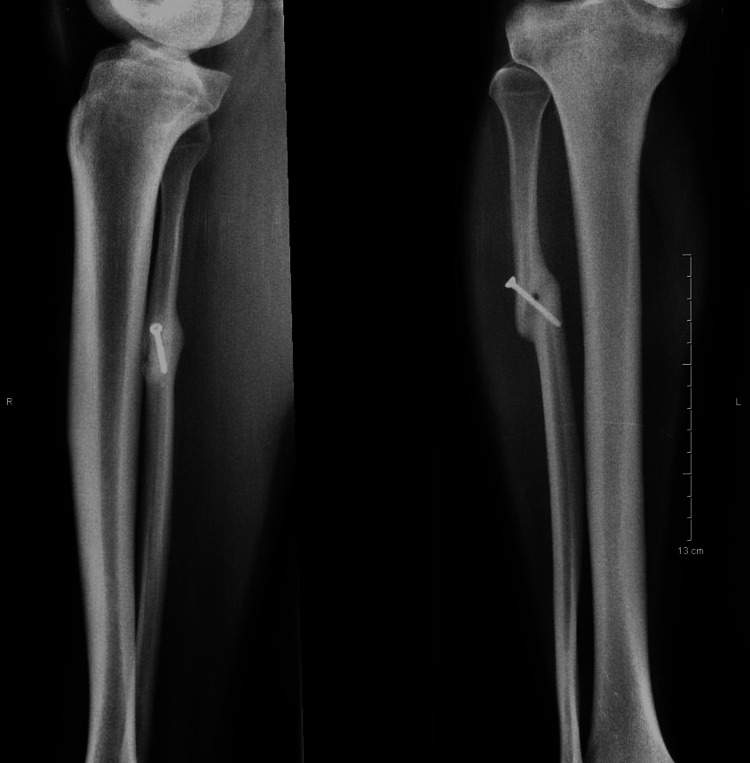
X-rays at eight weeks postoperatively depicting the complete union
